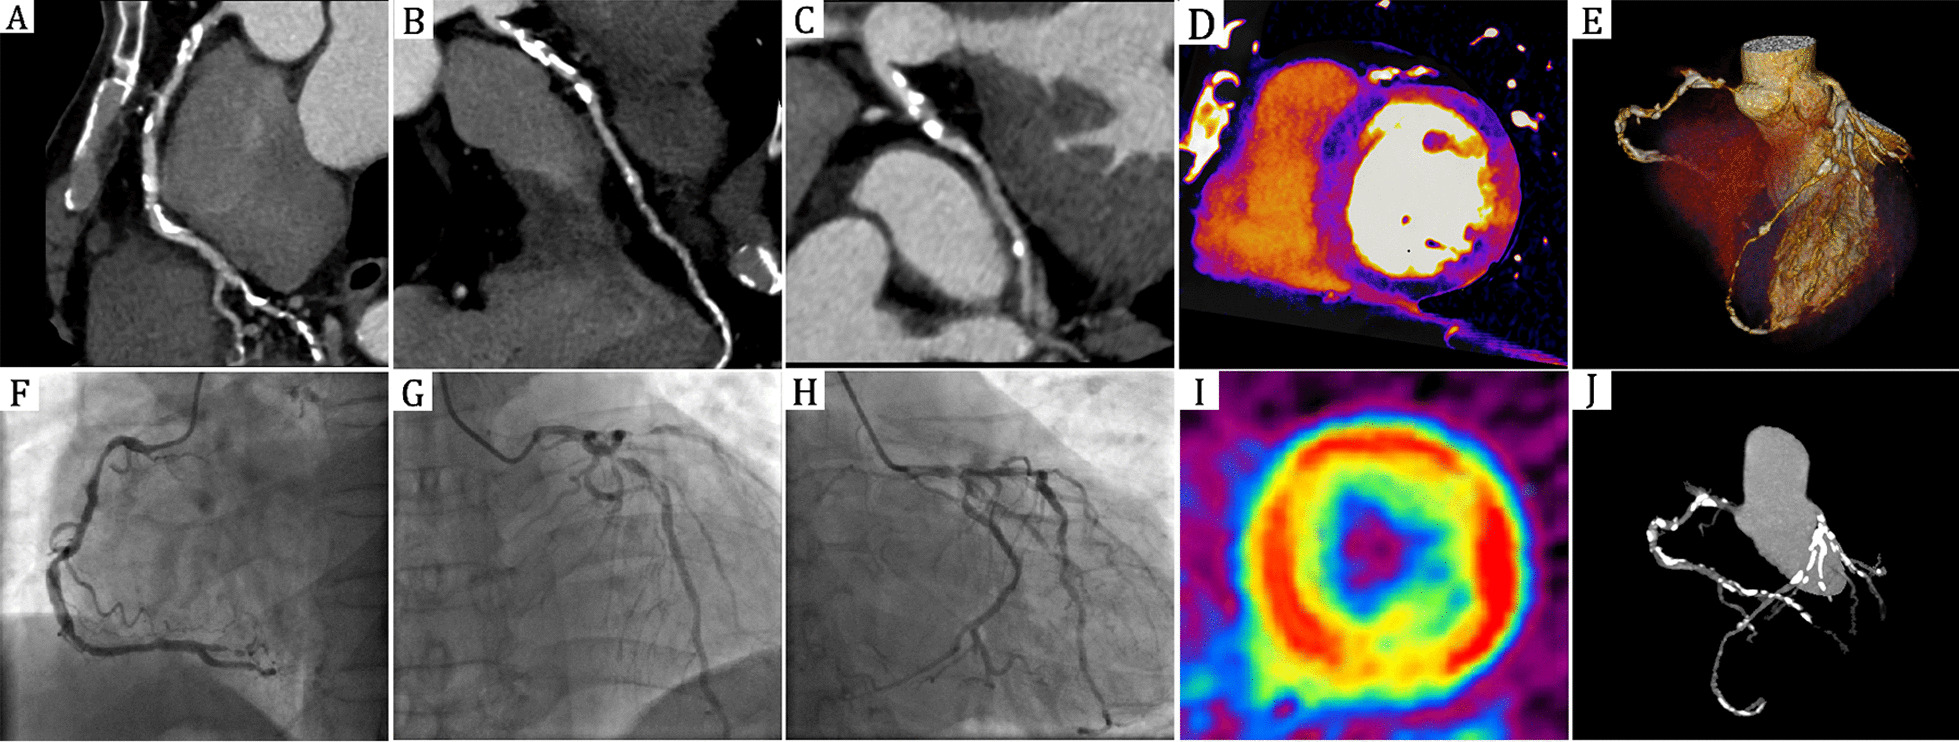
Match between coronary stenosis severity and resting DE-CTP. Curved multiplanar reconstruction CCTA reveals obstructive coronary artery disease with severe stenosis in RCA (A), LAD (B) and LCX (C). D Resting DE-CTP shows myocardial perfusion defects in anteroseptal, anterolateral, inferior walls and a subendocardial perfusion defect in inferolateral wall. This 63-year-old male subsequently underwent invasive coronary angiography (F–H) and PET (I) which confirmed the DE CT results. E, J are three-dimensional volume rendering technique (3D-VRT) and three-dimensional maximum intensity projection (3D-MIP) of CCTA respectively. DE-CTP = dual-energy CT perfusion; CCTA = coronary CT angiography; RCA = right coronary artery; LAD = left anterior artery, LCX = left anterior descending; PET = positron emission tomography
